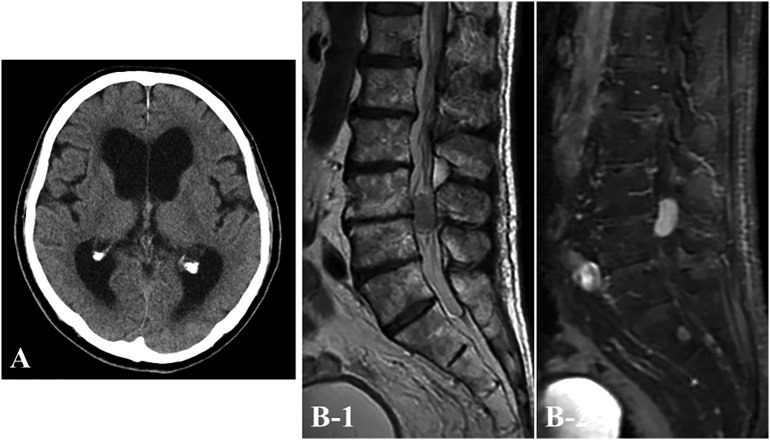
Preoperative computed tomography (CT) (A) shows a ventricular dilatation (Evans index, 0.4). Sagittal T2-weighted (B-1) and gadolinium (Gd)-enhanced T1-weighted (B-2) magnetic resonance imaging (MRI) reveal a homogeneously enhanced intradural extramedullary mass in the cauda equina at the L3-L4 level.
